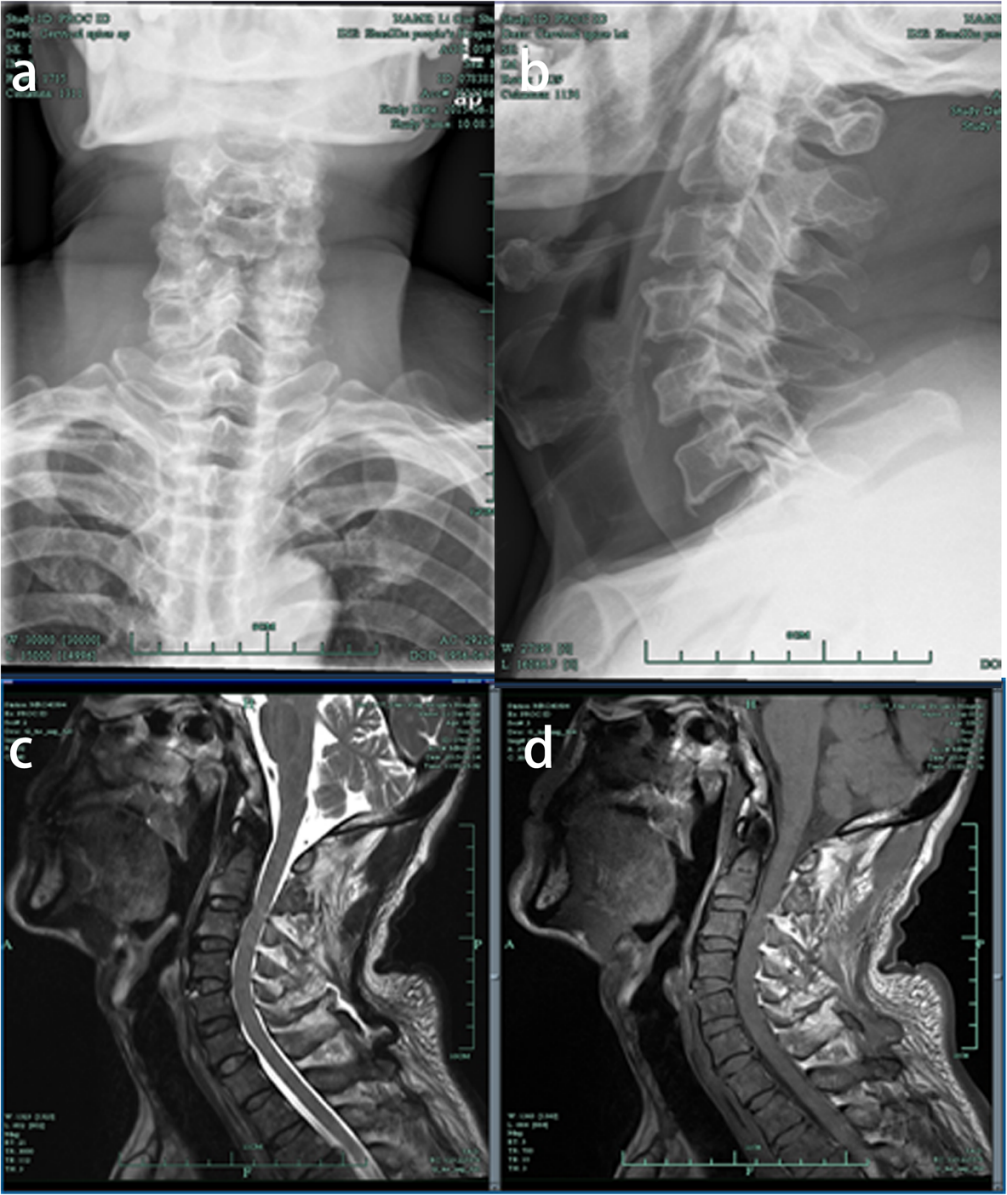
Imaging after first admission. a Anterior–posterior radiograph of the cervical spine demonstrating multilevel degenerative disc disease and facet arthropathy. b Lateral radiograph of the cervical spine showing an enlarged intervertebral space between C4–5 and degenerative changes of the spine. c T2-weighted sagittal image revealing anterior vertebral high signal and spinal cord signal changes at the C4/5 level. d There are no abnormalities in the throat
